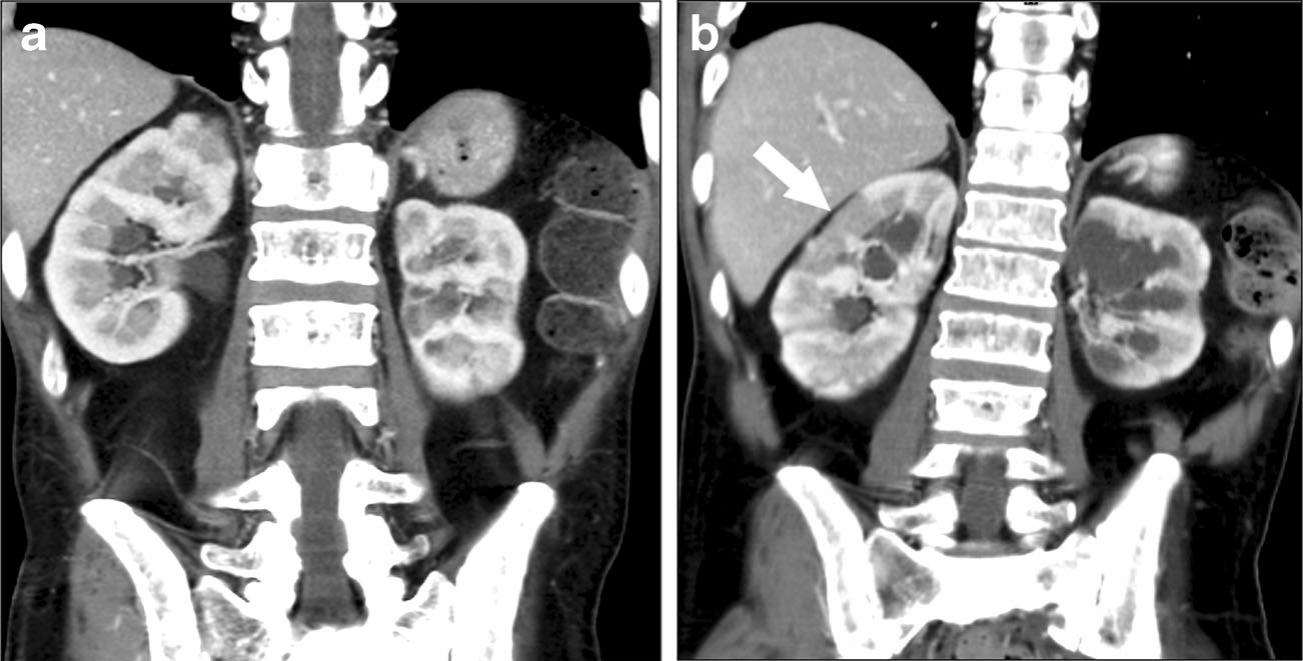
Forty-three-year-old male with soft tissue sarcoma, on a combination high-dose cisplatin chemotherapy regimen. (a) Pre-chemotherapy coronal CT showing normal renal findings. (b) Post-chemotherapy coronal CT showing an interstitial nephritis pattern with enlargement of the kidneys and multiple hypo-attenuating lesions, more prominent in the right kidney (*arrow*)
